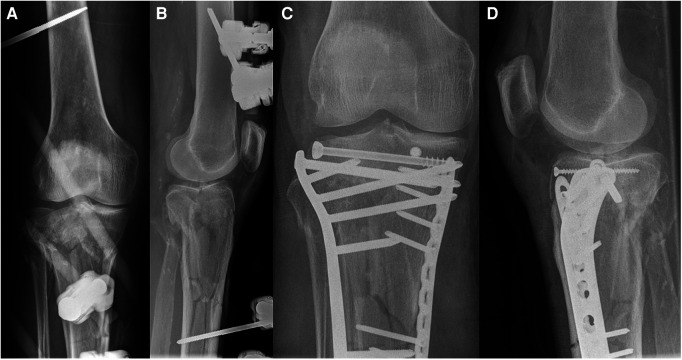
Case 3, comminuted 3-column fracture, AP (A) and lateral (B) x-ray after bridging external fixator (arterial injury ruled out by CT angiography), AP (C) and lateral (D) view after CRIF of articular fracture and MIPO of extraarticular fracture.
